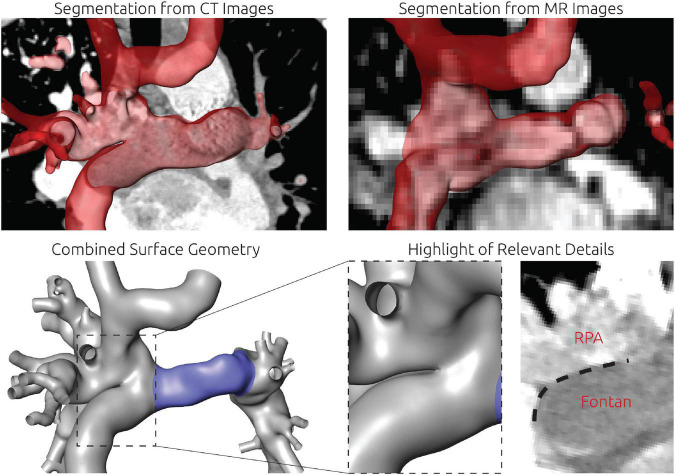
Detailed overview of the segmentation strategy for the first patient. The upper left and right panel show the segmentation from CT and CMR images, respectively. The lower left panel shows the final surface geometry that was reconstructed by combination of both segmentations. Tissue highlighted in blue was reconstructed from CMR images, whereas the remaining tissue was reconstructed from CT images. The lower right panel highlights the complex connection between RPA, SVC, and Fontan conduit, which could only be discriminated in CT images due to the distinct Hounsfield gradient from Fontan conduit to RPA.
